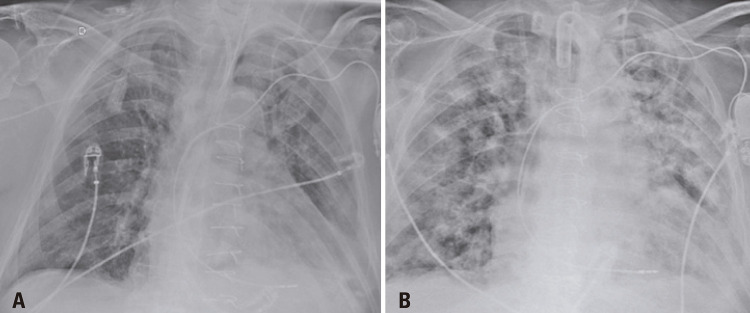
Bedside anteroposterior chest X-ray images demonstrate the radiographic evolution of the nosocomial infection with multiple areas of consolidation in both lungs
